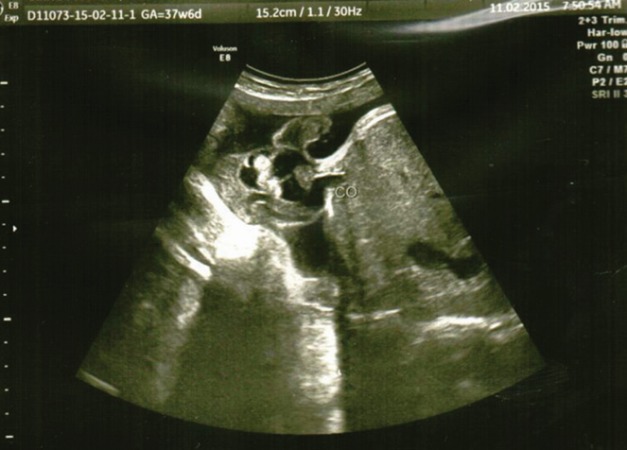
2D sonography–the insertion of the umbilical cord at the level of omphalocele to the viable fetus
